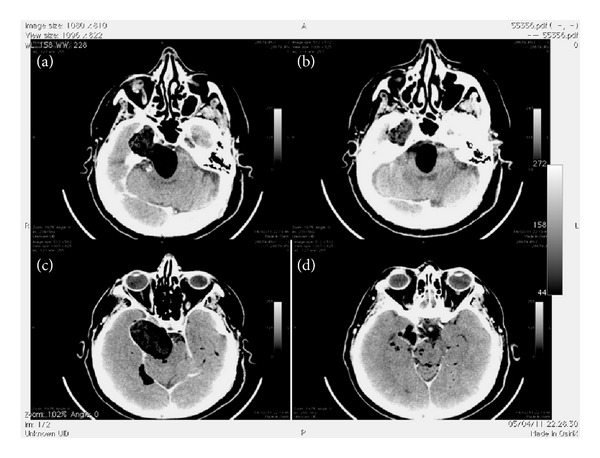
HRCT in axial plane showed heterogeneous hypodense lesion extending to middle and posterior fossa ((a) and (b)), and multiple bilateral hypodense collected disseminated along cerebral cisternal and cortical spaces ((c) and (d)).
